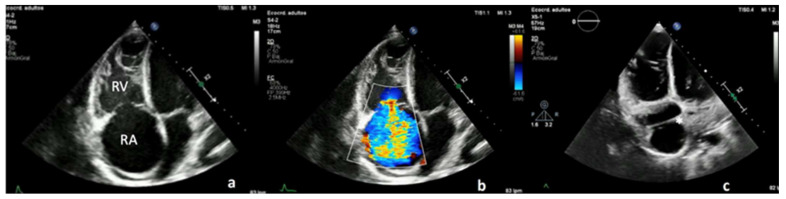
Transthoracic echocardiogram with severe right atrial (RA) and ventricular enlargement (a), severe tricuspid regurgitation (b) with an anomalous mobile membranous band-like structure crossing the RA (with asterisk in (c)). RV: Right ventricle RA: right atria.
